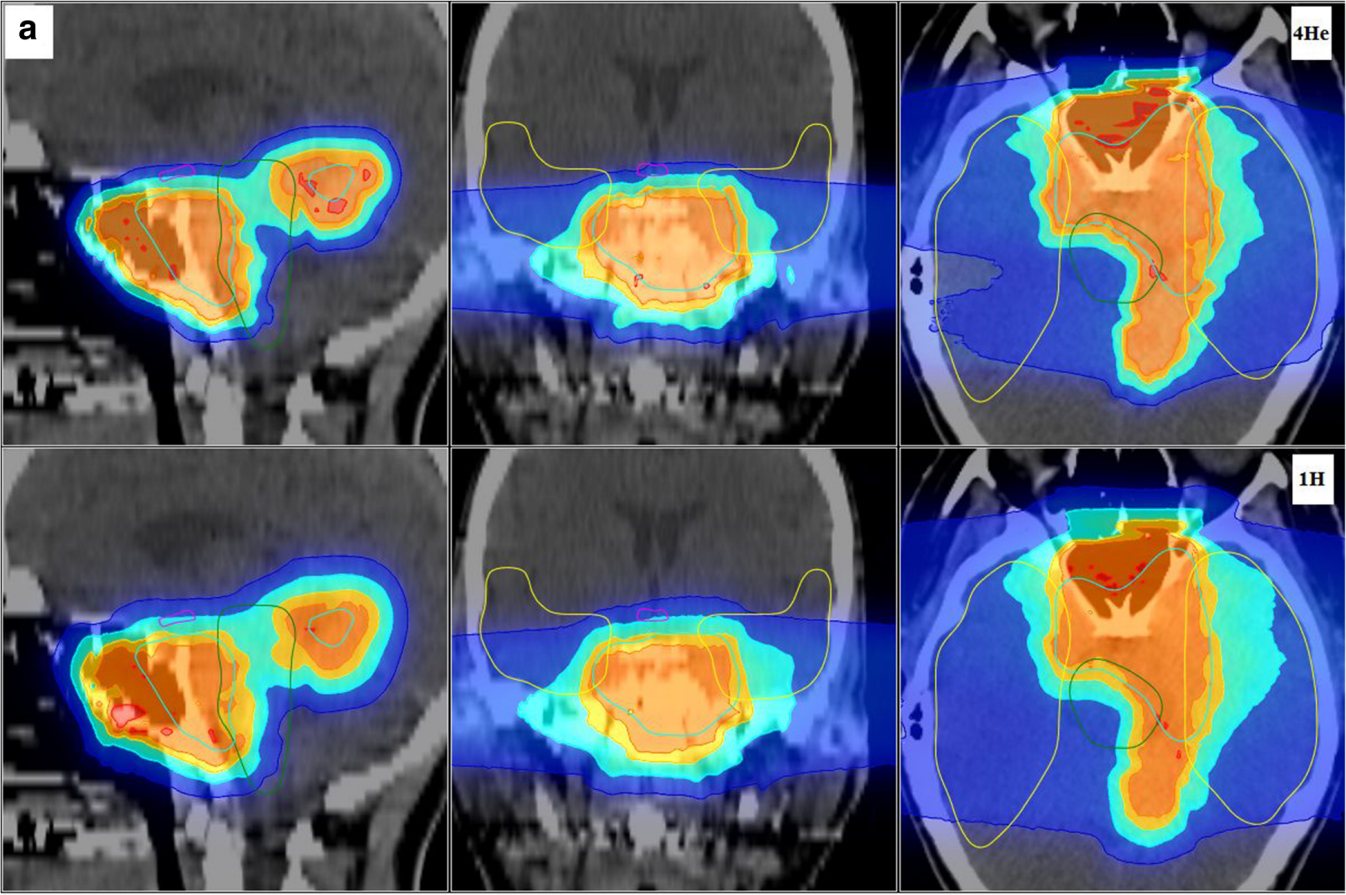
Planned dose distributions superimposed on the gray scale X-ray CT images are shown for patient A, featuring in the top panels helium ions (^4^He) and in the bottom panels protons (^1^H) for the sagittal (left), coronal (middle) and axial (right) slices
